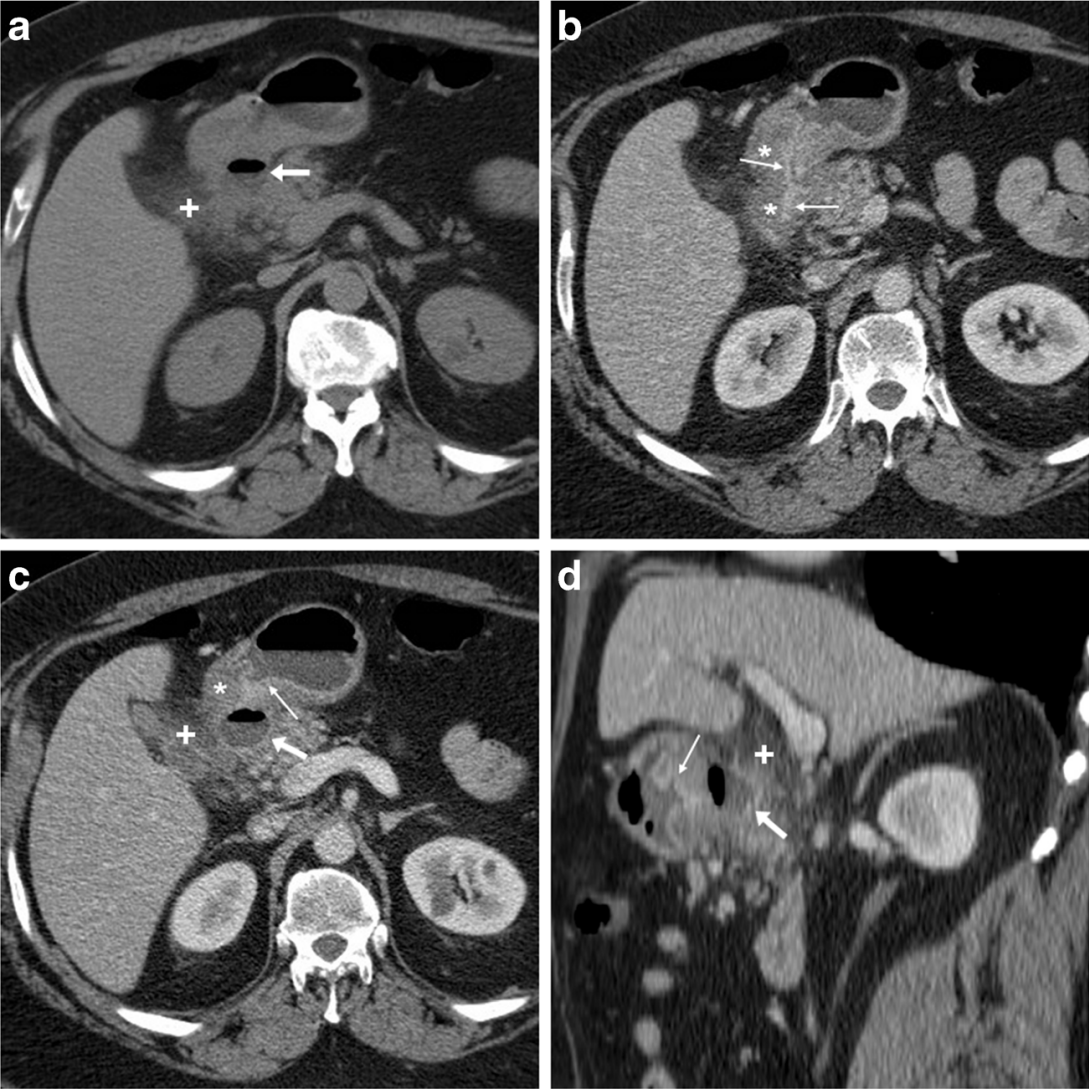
Three months later, the same patient in Fig. 1 experienced severe, recurrent abdominal pain with laboratory evidence of systemic inflammation (14.000/mmc leukocytes, 34 mg/L C-reactive protein). On further questioning, he admitted discontinuation of antacids and H2-blocker medications, and nonsteroidal anti-inflammatory drugs (NSAIDs) intake. Repeated CT revealed increased hypoattenuating mural thickening (*) with mucosal enhancement (thin arrows) of the pylorus and proximal duodenum which spared the anterior aspect, worsened periduodenal inflammatory changes (+), and development of a 2-cm roundish posterior deep ulcer (arrows). Upper digestive endoscopy confirmed a non-malignant retropyloric peptic ulcer. The patient ultimately did well on proton-pump inhibitors (PPI) and anti-*Helicobacter pylori* triple therapy [Adapted from Open Access ref. no [10]]
